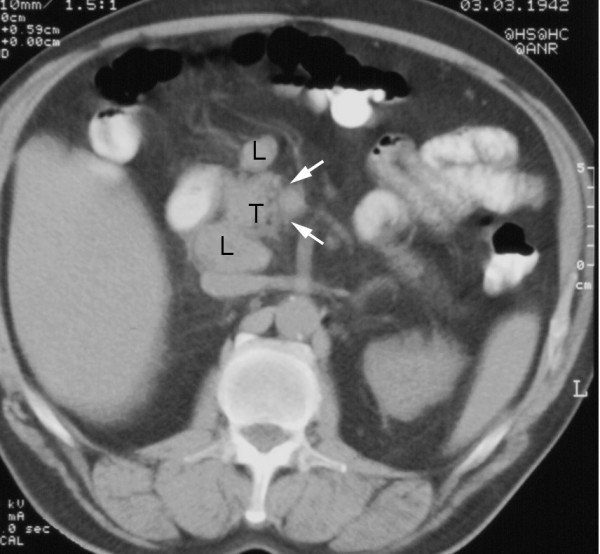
CT at time of diagnosis before chemotherapy. T = tumor; L = lymph node; arrows indicating semicircular contact to the superior mesenteric vein.
